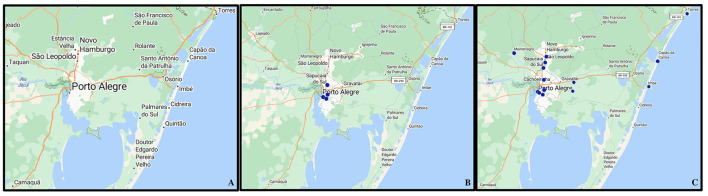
Acute stroke network in the region. Blue dots in the map represent stroke centers available in each period. (A) Before stroke unit (BSU—Baseline Scenario): twenty-six hospitals with CT capability managed all stroke patients, who were treated in general emergency departments and admitted to general wards. There were no dedicated stroke centers in the region. (B) Acute stroke unit (ASU—Pilot Phase): four hospitals, designated as acute stroke centers and participating in a Ministry of Health pilot program, received all stroke patients in the region. (C) Comprehensive stroke unit (CSU—post-policy implementation): following the implementation of the national stroke policy, 16 stroke centers with dedicated stroke units (acute + rehabilitation) were established, enabling a regionalized distribution of stroke patients across the network.
